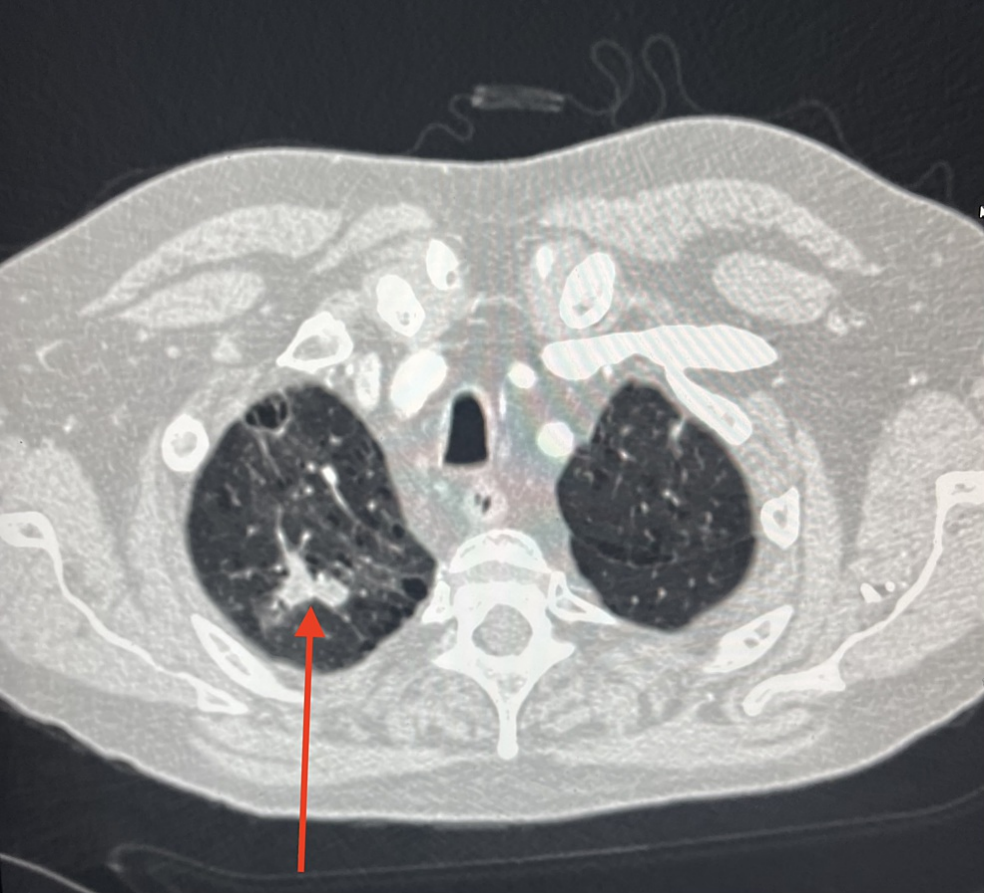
CTA of the chest displaying a 3×2 cm spiculated consolidation in the right upper lung lobe. CTA: CT angiography
